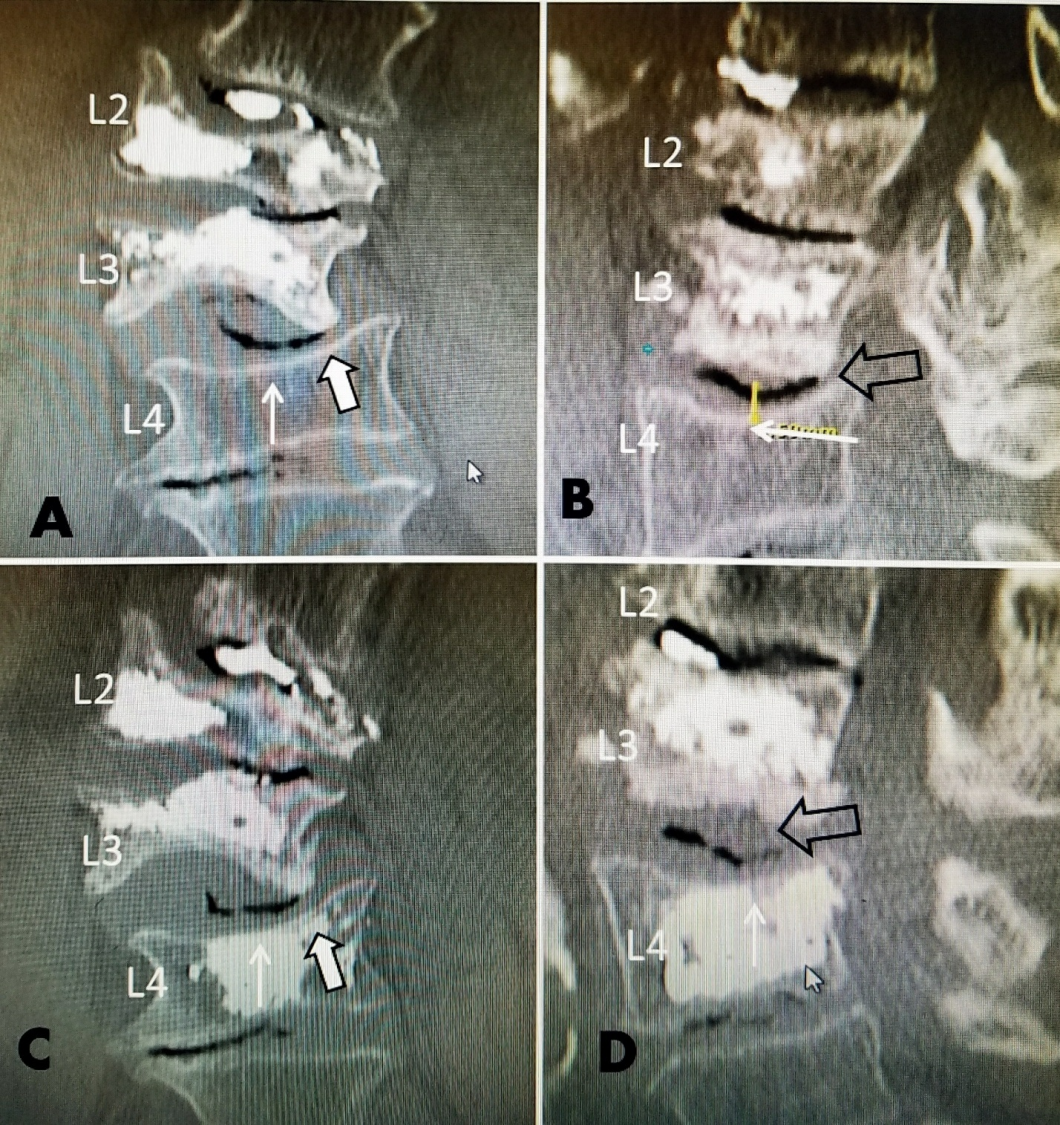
A 78-year-old female with previous kyphoplasty at L2 and L3 with progressive right convex scoliosis and a fracture at L4 A: Coronal computerized tomography (CT) showing a superior endplate collapse at L4 (solid white arrow). The edge of the inferior endplate of L3 is pressing into the depressed superior endplate of L4 before the actual development of an acute fracture (solid, large white arrow with black border). There are multiple vacuum disc defects at L2-3, L3-4, and L4-5. The angled L3 vertebra is collapsing into the superior endplate fracture of L4 (solid, large white arrow with black border). B: A lateral CT scan showing superior endplate subsidence without a clear fracture at L4 (solid white arrow). The bone scan showed mild uptake at L4. The disc space height between L3 and L4 is normal (yellow arrow). There is a large vacuum cleft sign across the entire L3-4 disc space (open black arrow). C: Coronal CT scan after kyphoplasty combined with the insertion of a Kiva polyethyletherketone implant PEEK-OPTIMA (Benvenue Medical Santa Clara, California, USA) at L4. The cement and PEEK-OPTIMA cage was placed directly under the center of L4 ( small white arrow), which is the downward pressure point from the shifted L3 vertebra (large, solid white arrow with border). D: Sagittal CT scan after L4 kyphoplasty with a Kiva system and polymethyl methacrylate cement (PMMA). The cement is filling the entire peek cage placed transpedicularly, providing strong support under the collapsing superior endplate of L3 (solid white arrow). Note that the vacuum cleft previously seen in the L3-4 disc space is much smaller (open, large black arrow). This was performed with the patient's consent as a preventive kyphoplasty because of the lateral instability and angulation of the L3 inferior vertebrae into L4 on the coronal views. The patient did not develop further fractures over a 24-month follow-up.
